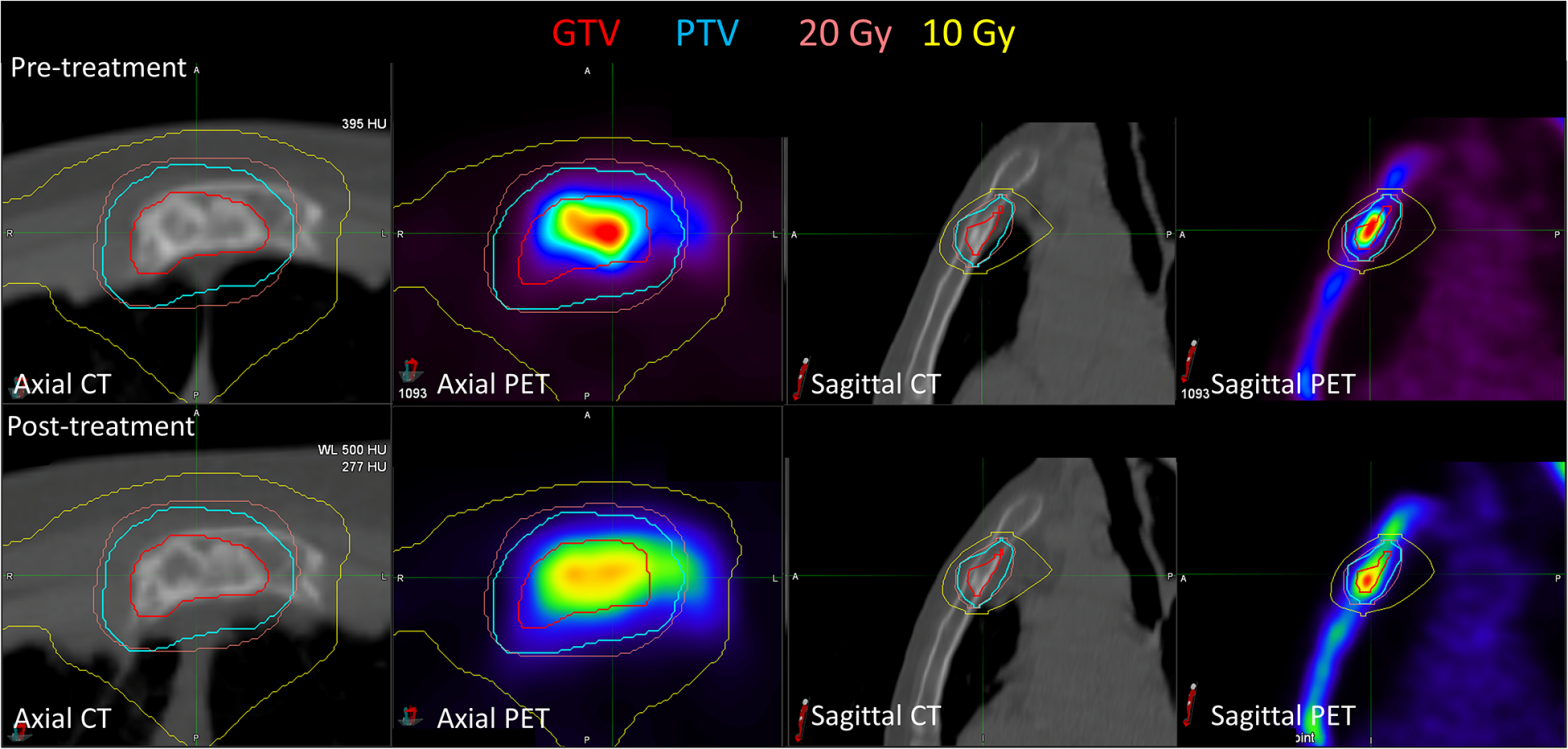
Pre-treatment and post-treatment CT and [^18^F]NaF PET for patient 13, who had a sternal metastasis treated. Both SUV_max_ (decreased from 18.0 to 17.0) and SUV_mean_ (increased from 8.7 to 9.5) were relatively stable post-treatment. There were minimal observable CT changes consistent with minimal bone regeneration.
